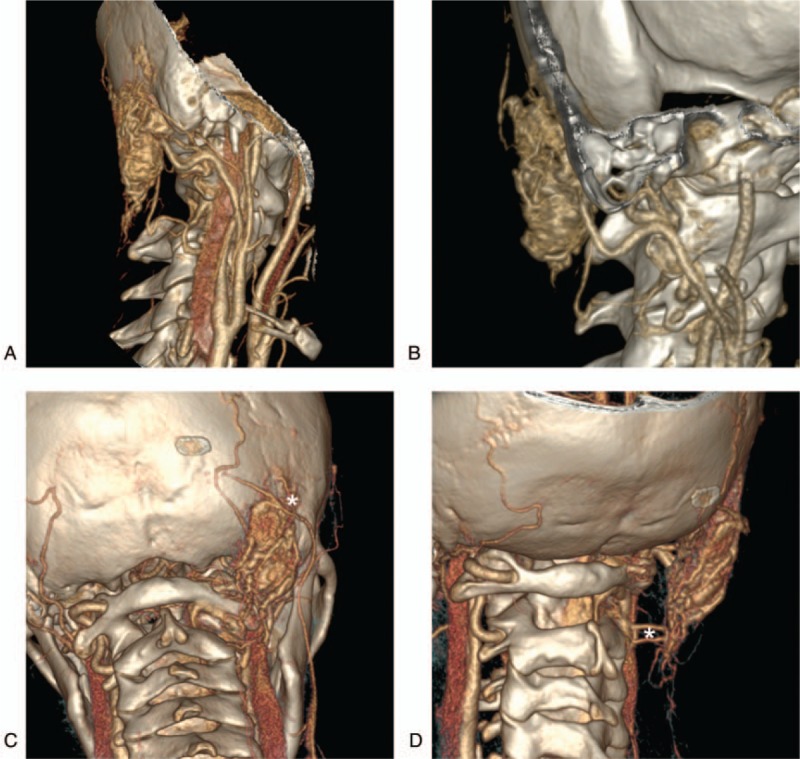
Preoperative CTA images. A–B: CTA after the reconstruction revealed the main blood supply arteries from multiple angles. The lesion size was approximately 3 × 5 cm, and it was mainly supplied by the occipital artery and the muscle branch of the vertebral arteries. C: The venous drainage of the lesion communicated with the suboccipital venous plexus at the anterior and posterior CTA, and it also communicated with the subcutaneous superficial vein. D: The venous drainage of the lesion communicated with the paravertebral venous plexuson anterior and posterior oblique CTA (white asterisk). CTA = computered tomography angiography.
